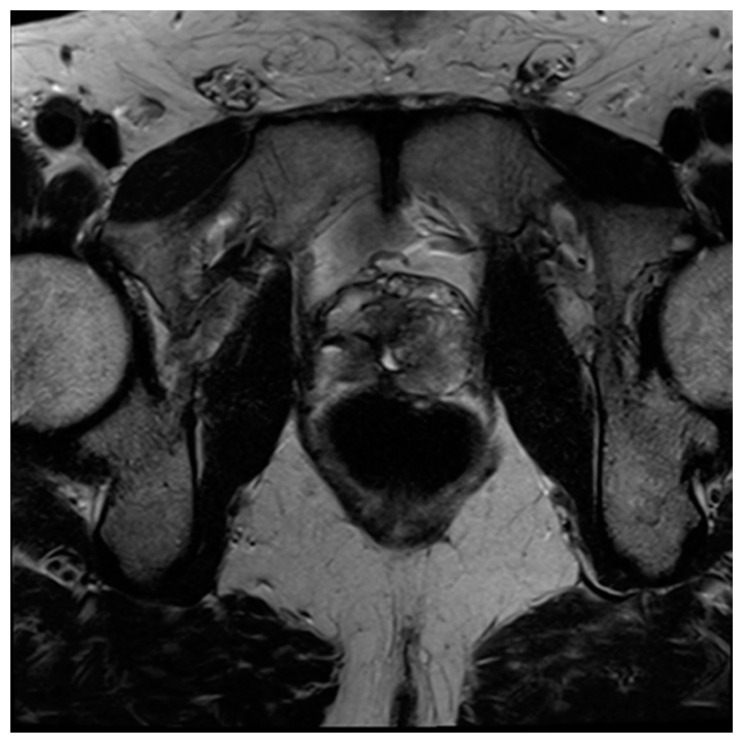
Post-HIFU ablation defect seen on axial T2 MRI as asymmetric atrophy with adjacent fibrosis and architectural distortion, predominantly involving the right hemigland.
